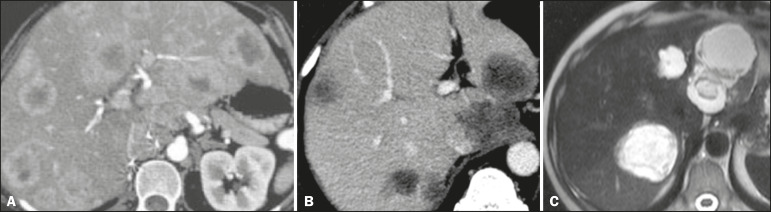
Possible differential diagnoses for metastases from an NEN. Liver metastasis from a renal cell carcinoma—arterial phase CT scan (A), from adenocarcinoma of the colon—arterial phase CT scan (B), and from sarcoma—T2-weighted MRI sequence (C).
